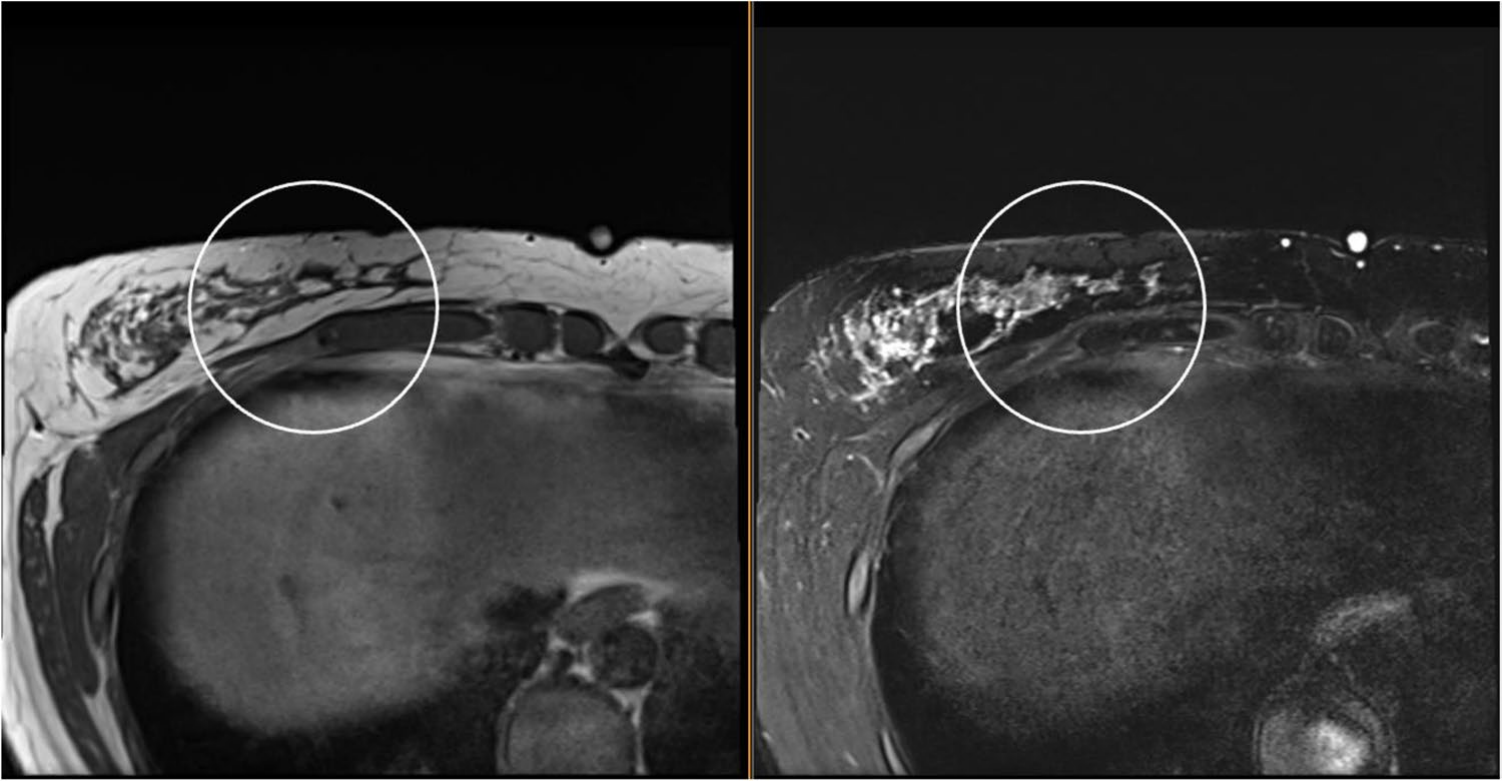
Follow-up surveillance MRI of the chest without contrast approximately 4 months after radical resection of the right chest wall mass shows no mass or signal abnormality to suggest residual or recurrent disease (circle denotes area of previous mass)
